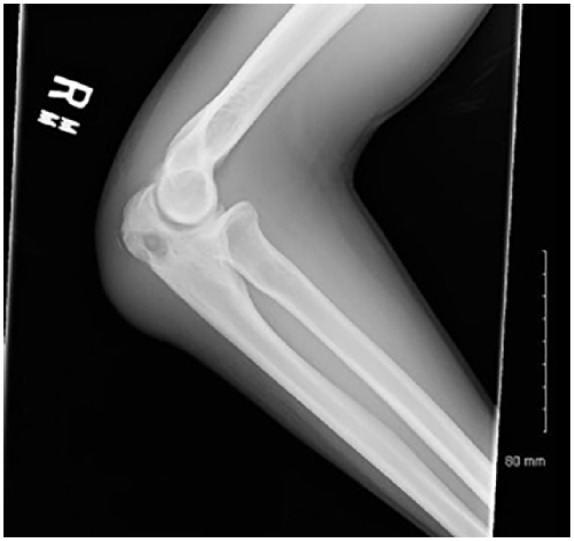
Right elbow X-ray on admission.
